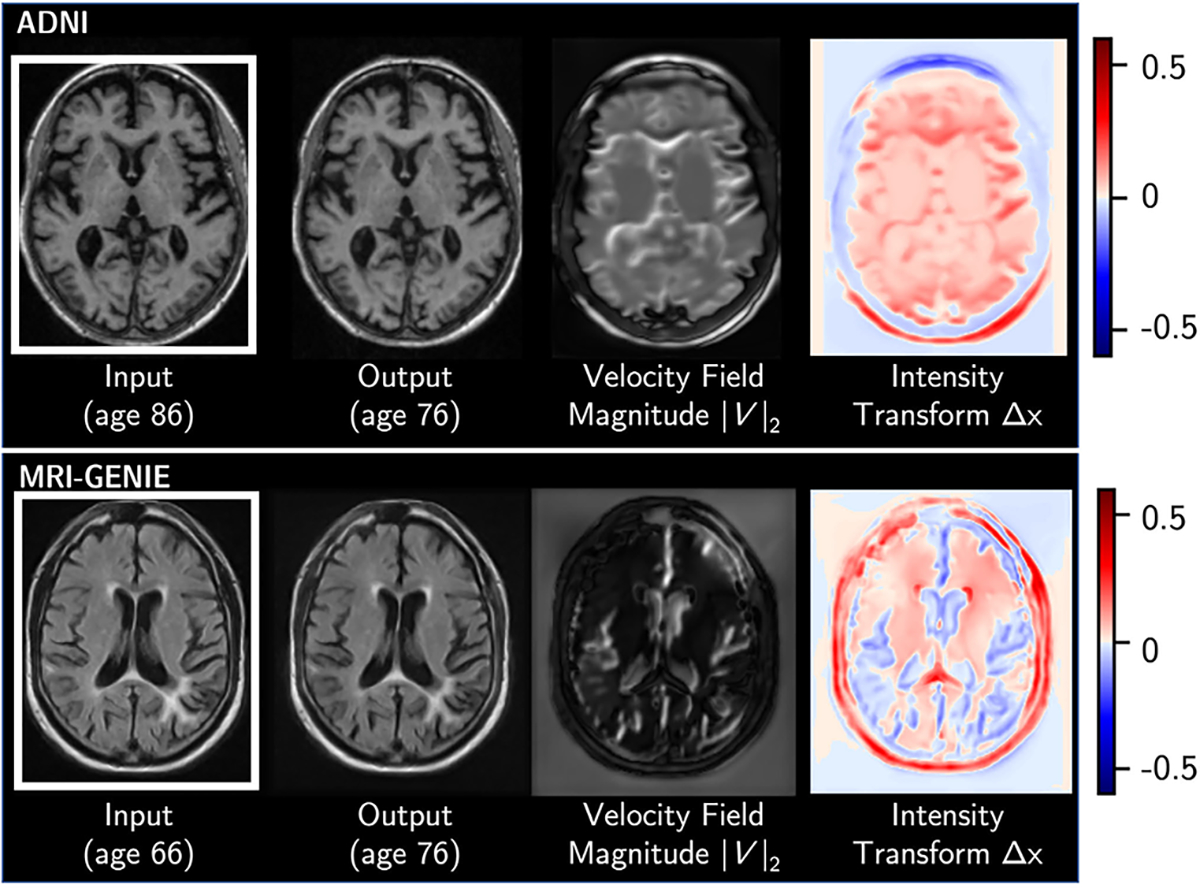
An example of the spatial and intensity transforms produced by SIT-StarGAN for an age-conditioned translation in ADNI *(top)* and MRI-GENIE *(bottom)*.
